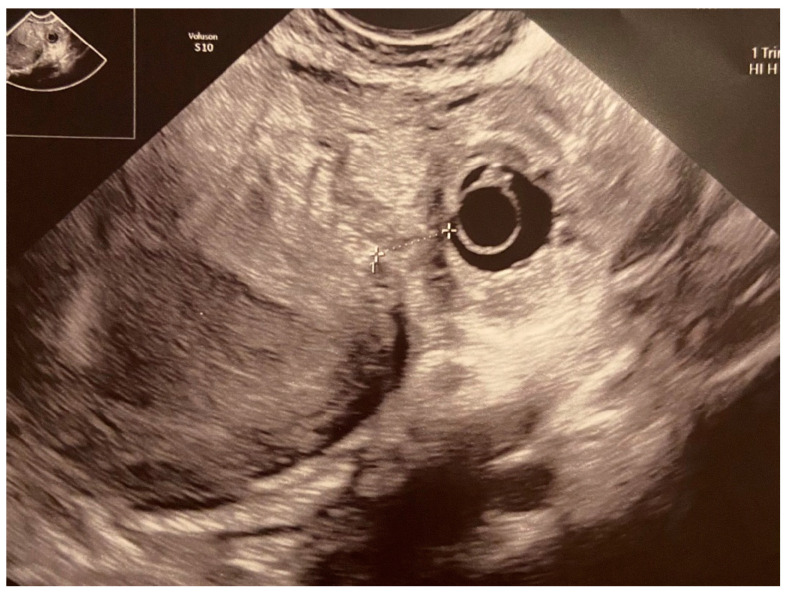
Patient B—2D transvaginal ultrasound of IP within the left uterine horn, cornual bulge.
